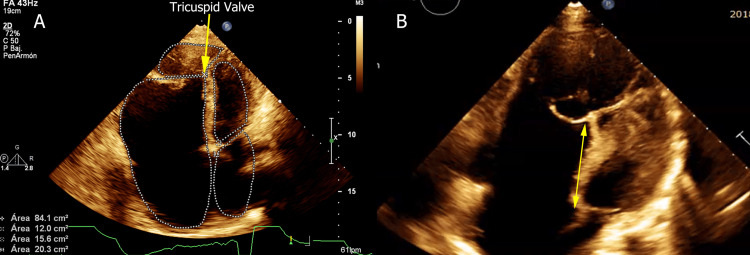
Transthoracic echocardiography. (A) Apical four-chamber view showing low (apically displaced) tricuspid implantation, right atrial dilation, and a small functional right ventricle, with intact interatrial and interventricular septa. (B) Absent delamination of the anterior tricuspid leaflet with right atrial dilation.
